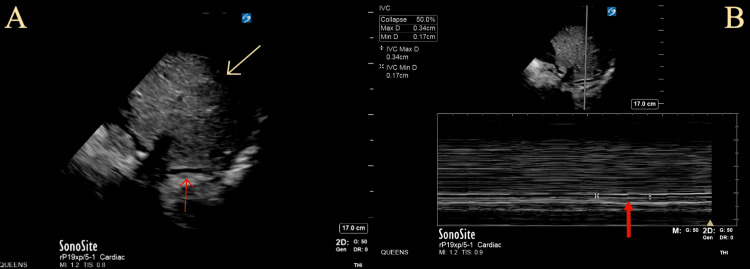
Point-of-care ultrasound with (A) subxiphoid view demonstrating the inferior vena cava (red arrow) and liver (yellow arrow), and (B) collapsed inferior vena cava (red arrow) with a maximum and minimum diameter of 0.34 cm and 0.17 cm, respectively.
